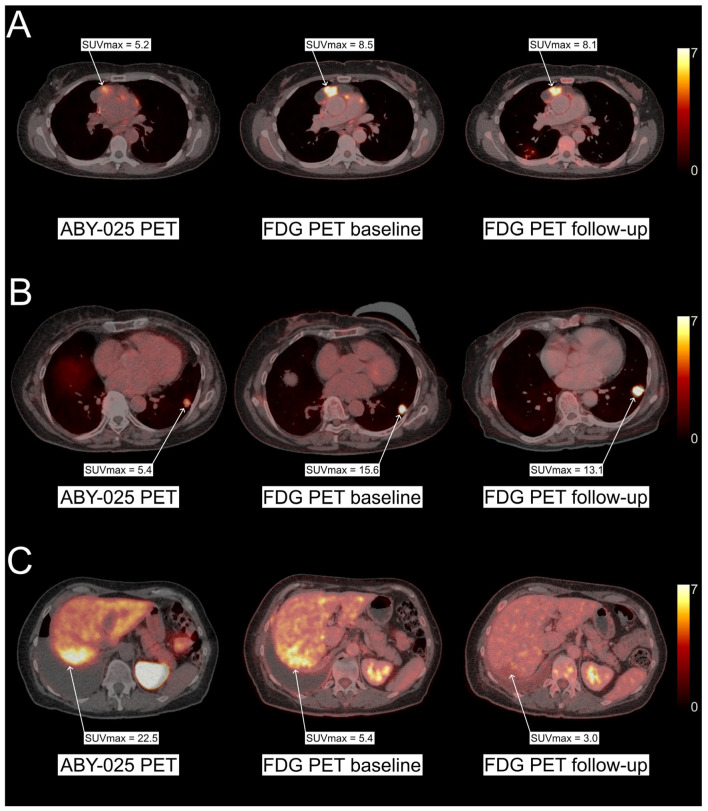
Representative images of PET scans conducted in the Affibody-3 trial (NCT03655353) provided by Jens Sörensen and colleagues. (A) (pt3): Breast cancer (BC) patient with biopsy-confirmed HER2-positive mediastinal metastasis (biopsy results: IHC2+/ISH+), [^68^Ga]Ga-ABY-025 uptake at baseline was low (SUVmax = 5.2). No metabolic response was observed after receiving two cycles of HER2-targeted therapy (B) (pt5): BC patient with biopsy confirmed HER2-positive lung metastasis (biopsy results: IHC3+/ISH−) with low [^68^Ga]Ga-ABY-025 uptake at baseline (SUVmax = 5.4). The patient had disease progression despite receiving three cycles of HER2-targeted therapy. (C) (pt15): BC patient with biopsy confirmed HER2-negative liver metastasis (biopsy results: IHC1+/ISH−). [^68^Ga]Ga-ABY-025 PET showed high uptake in tumor lesions (SUVmax = 22.5), and the patient showed a good metabolic response after two cycles of HER2-targeted therapy. Therapies received during the study period: Patient 3: epirubicin + cyclophosphamide + trastuzumab + pertuzumab; Patient 5: trastuzumab emtansine (FDG-PET after three cycles in this patient); Patient 15: docetaxel + trastuzumab + pertuzumab.
